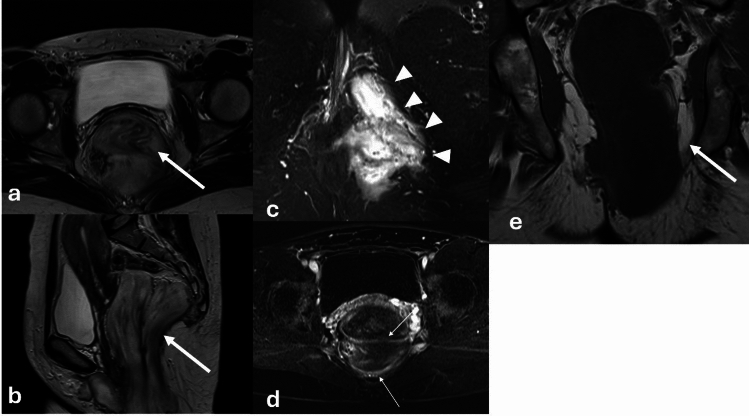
51-Year-old female without significant past medical history presenting with approximately 1 year of pelvic and perineal fullness, more apparent during exercise. Two months prior to presentation, the patient noticed a bulge in the left vulvar region and new onset constipation which prompted an OB/GYN appointment. A palpable mass was confirmed on physical exam and a pelvic MRI was obtained which showed an approximately 15 cm bilobed mass in the low pelvis between the rectum and vagina extending through the urogenital diaphragm and into the left perineum. The mass demonstrated homogeneous low signal on T1, high signal on T2 with swirled low intensity bands which enhanced on post contrast imaging. The patient was treated preoperatively with GnRH analog injections in an effort to shrink the mass followed by surgical debulking. Pathology confirmed aggressive angiomyxoma. Axial (a) and sagittal (b) T2-weighted images of the pelvis demonstrate a T2 hyperintense lesion (arrow) with swirling T2 hypointense internal striations centered in the rectovaginal space, traversing the urogenital diaphragm and extending inferiorly to the left perineum. Perineal extent is best appreciated on fat-saturated axial T2-weighted images (c) (arrowheads). The internal striations demonstrate modest enhancement (thin arrows) on axial post-contrast T1 subtraction images (d). On coronal T1-weighted images (e), the lesion is uniformly hypointense
